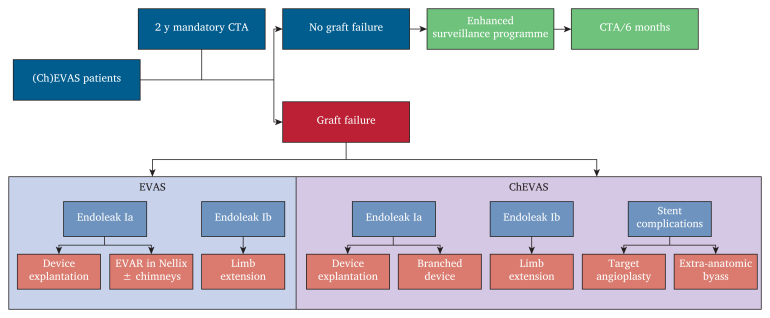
Management strategy for patients treated with endovascular aneurysm sealing (EVAS) and chimney grafting in combination with endovascular aneurysm sealing (ChEVAS). CTA = computed tomography angiography.
